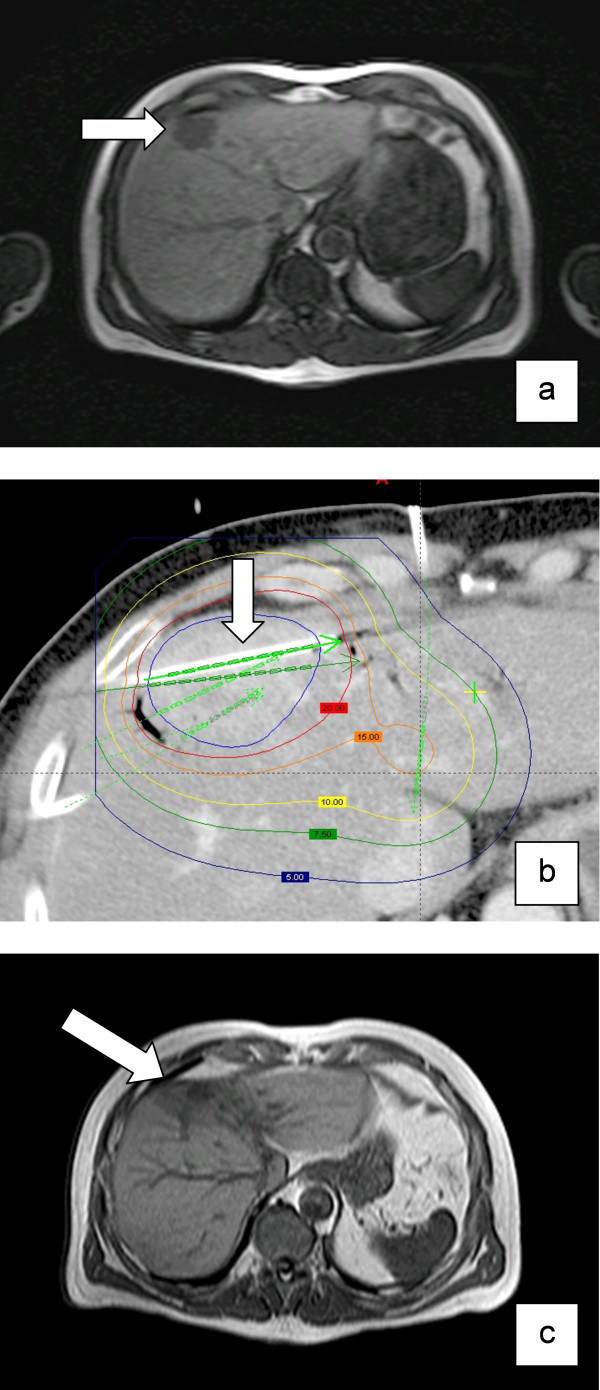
Development of radiation injury of the liver after HDR brachytherapy: (a) Colorectal metastasis in liver segment IV (arrow), T1w-GRE 20 minutes after application of Gd-BOPTA. (b) Contrast-enhanced planning-CT and dosimetry after insertion of brachytherapy catheters (arrow) in the metastasis. The coloured lines indicate different isodoses. Applicated dose at the tumor margin was 20 Gy. (c) MRI after 3 months with a decreased uptake (arrow) of Gd-BOPTA around the irradiated and shrunken metastasis (T1w-GRE 20 minutes after application of Gd-BOPTA).
